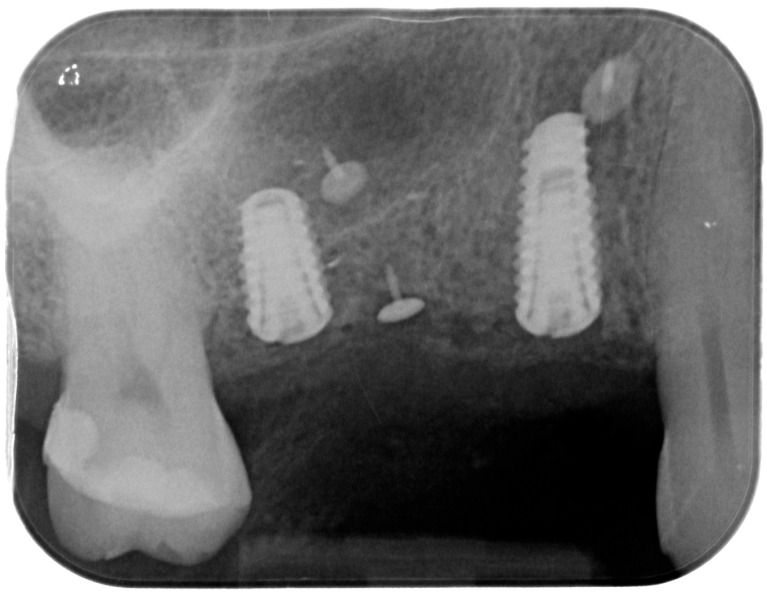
Postoperative intraoral X-ray.
